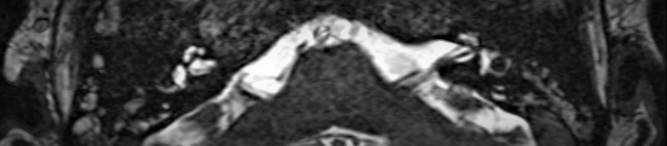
Preoperative axial magnetic resonance imaging (T2 CISS3D): no signs of cochlear obliteration
